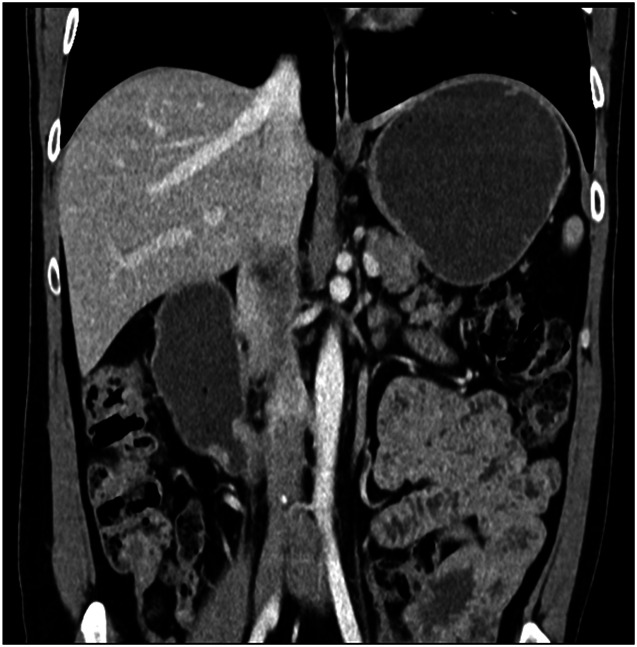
Computed tomography scan showing dilation of the stomach and duodenal bulb with an abrupt narrowing at the duodenal sweep.
